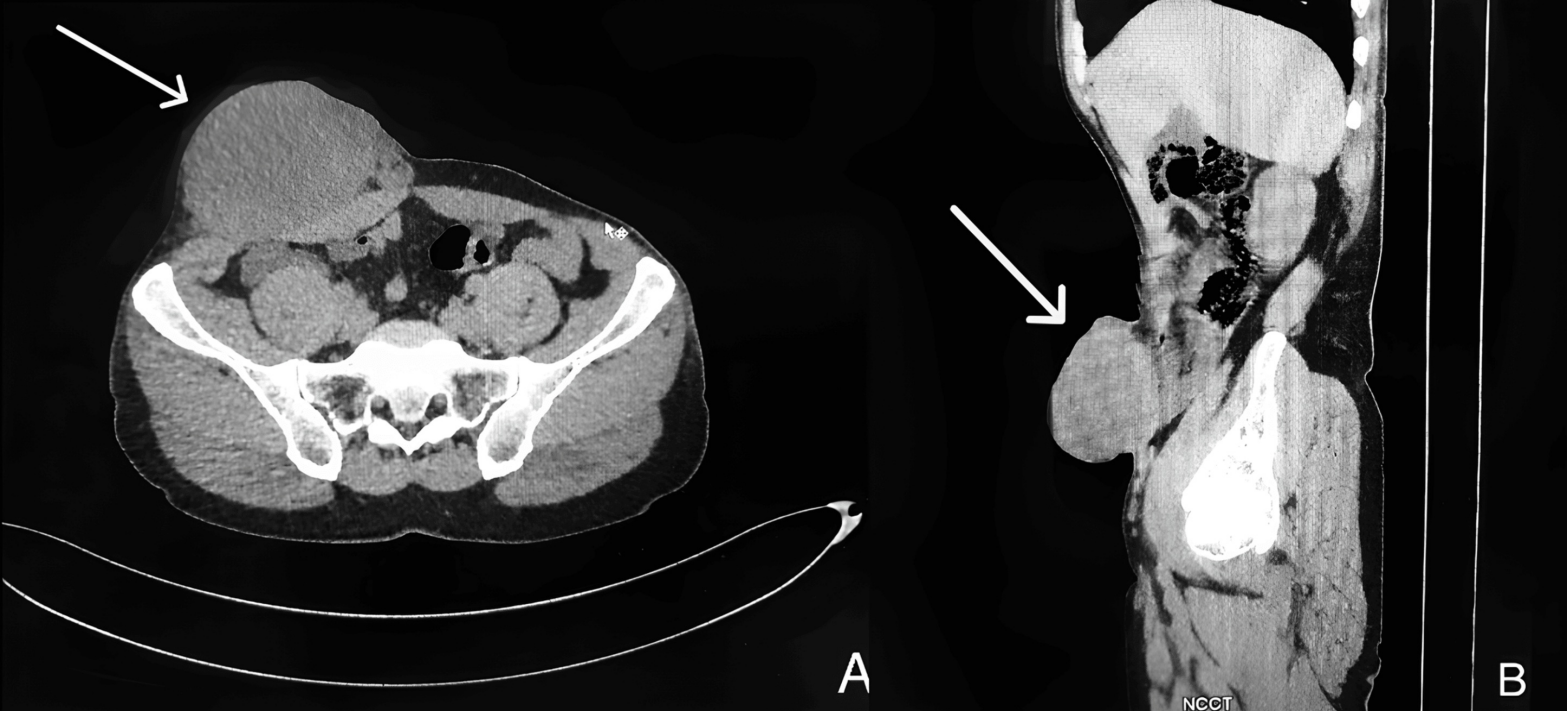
Axial and sagittal sections of plain CT showing a multilobulated, heterogeneously enhancing soft tissue density lesion, located in the subcutaneous planes of the right lower anterior abdominal wall, with no obvious intra-abdominal extension.
